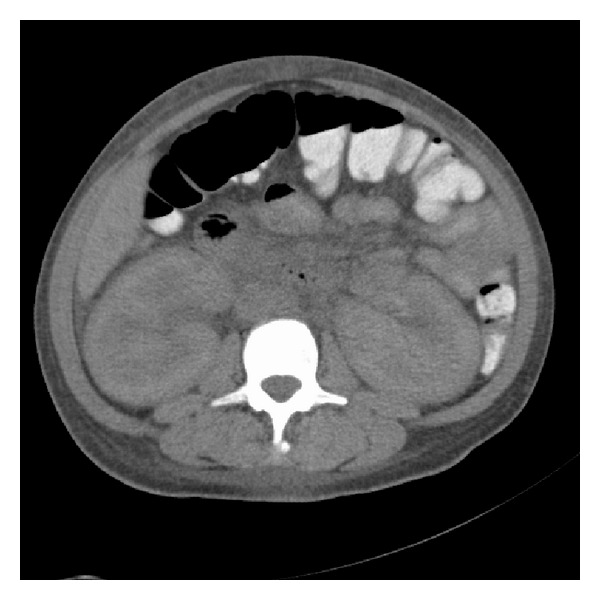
Computed tomography (CT) scan of the abdomen/pelvis without contrast revealing bilateral enlarged and edematous kidneys.
